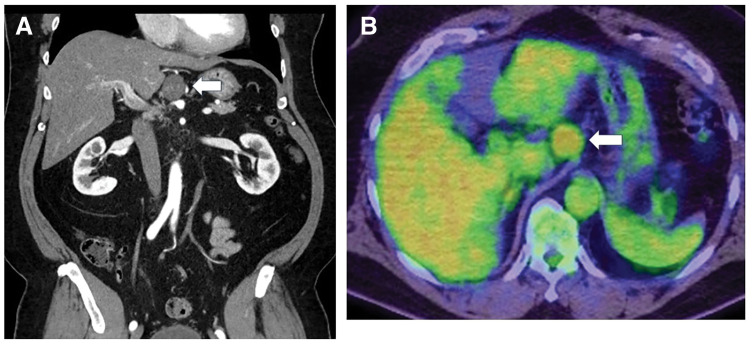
(A) An abdominal dynamic contrast-enhanced CT scan showed a 25-mm well-defined, hypodense tumor (arrow) adjacent to the left gastric artery. (B) PET-CT detected the tumor with an SUVmax of 2.3 (arrow), without evidence of distant metastases. SUVmax, maximum standardized uptake value
